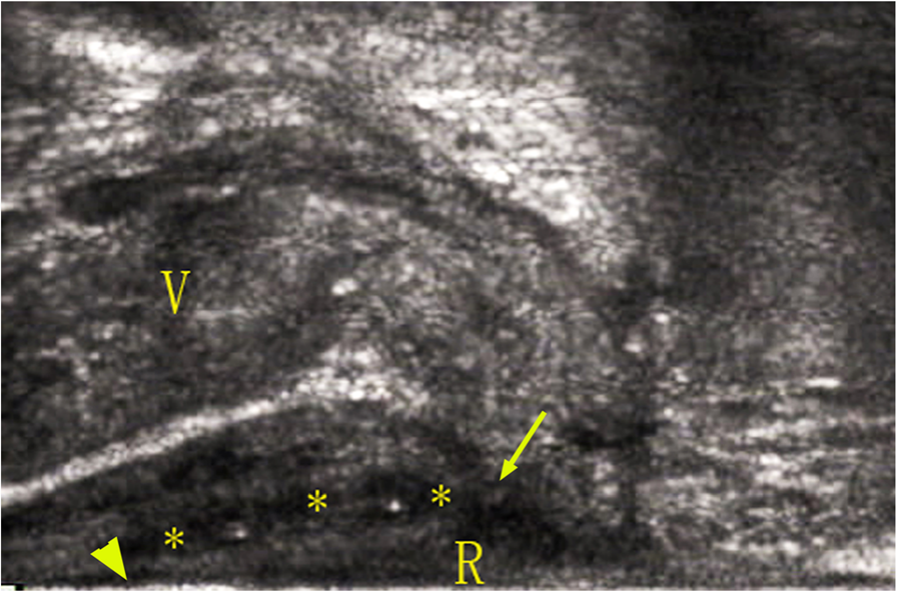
RVF morphology observed using a linear array probe on transrectal sagittal section. The ultrasonic image clearly demonstrates the fistula opening at the vagina and fistula opening in the rectal wall using the linear array probe, which is more advantageous than the convex array probe when observing fistula morphology and the surrounding anatomical structures. Abbreviations: V, vagina; R, rectum. The small arrow indicates the fistula opening at the vagina. The large arrow indicates the fistula opening in the rectal wall. The asterisks denote the fistulas, in which punctate strong echoes formed by gas can be seen
